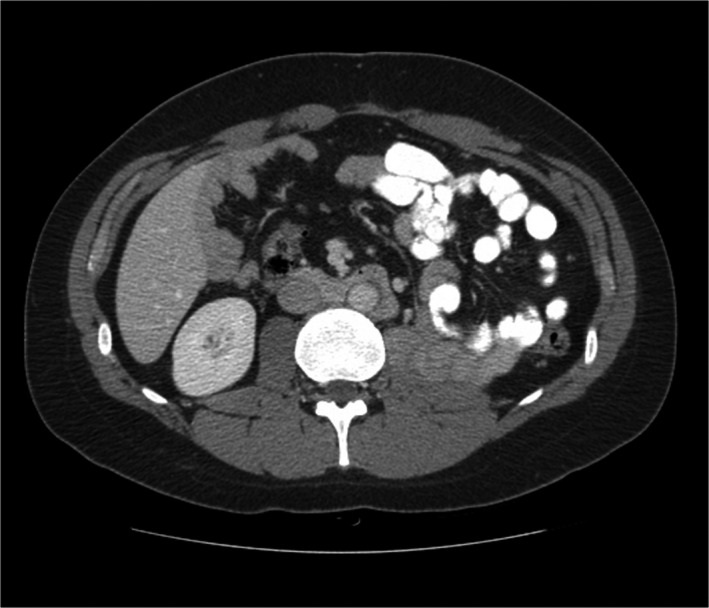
Representative image from an abdominal CT scan on 05/06/2013 showed no evidence of new mass lesion or local recurrence
